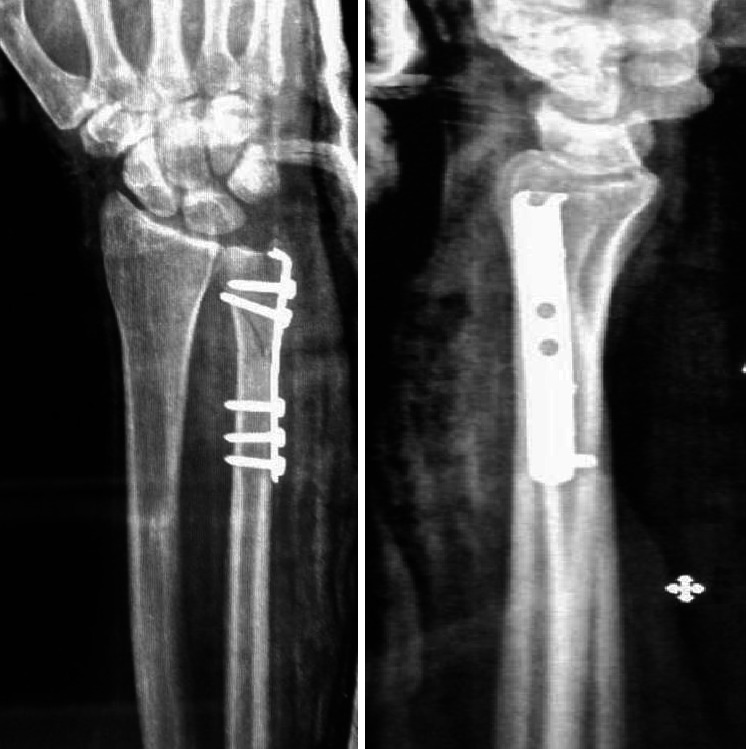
Postoperative X-ray wrist anteroposterior and lateral views show the fracture after fixation by a 2.7 mm semitubular plate.
